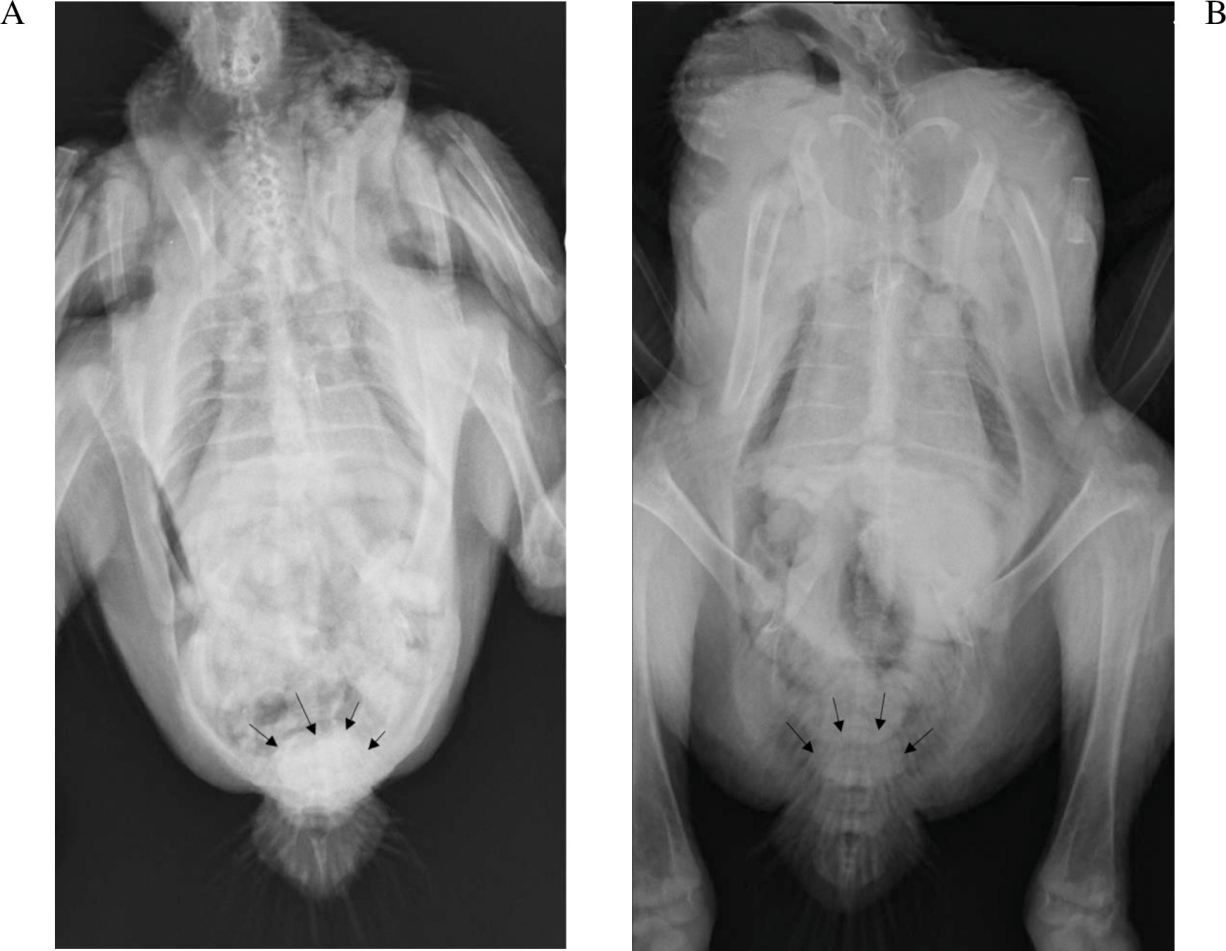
Radiographs of chicken 9192 five minutes after iohexol administration. The iohexol is more dense in the cloaca (arrows) in the absence of diclofenac (A) than with (B).
